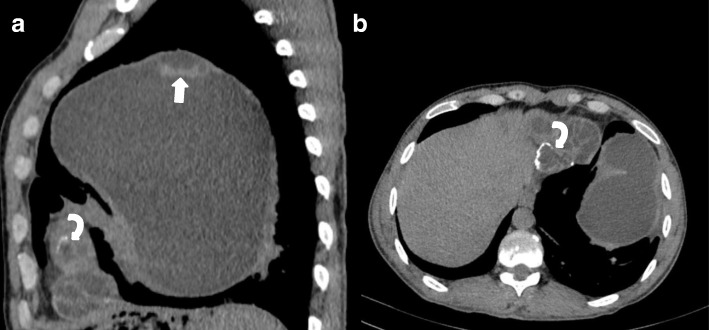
a-b: Unenhanced chest CT sagittal and axial sections: a large, well defined, fluid attenuating, non-enhancing cystic mass lesion in the left lung mostly occupying the left lung upper lobe. Some internal septations are seen in the superior aspect of this cystic lesion (Arrow). Parts of a multi-Septate, fluid attenuating cystic lesion is also seen in the cardiac apex with peripheral wall calcification (curved arrow)
